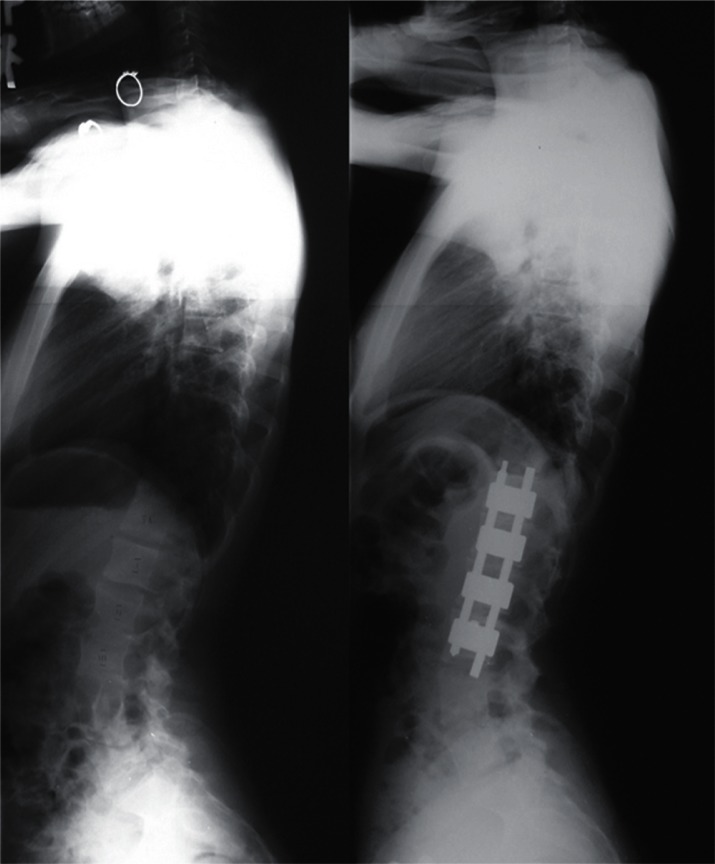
Anterior instrumentation surgery. Lateral radiographs before and after surgery
